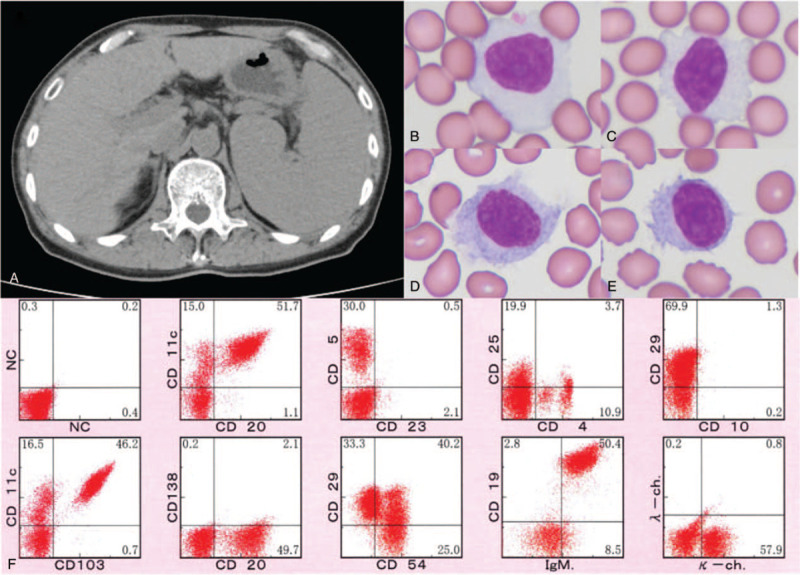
Imaging and laboratory findings during the second relapse. (A) Computed tomography shows splenomegaly. (B, C) Instrument-dried peripheral blood shows atypical cells with abundant cytoplasm. (D, E) Naturally dried peripheral blood shows villous cells with prominent nucleoli. (F) Flow cytometry analysis of peripheral blood after rituximab treatment.
